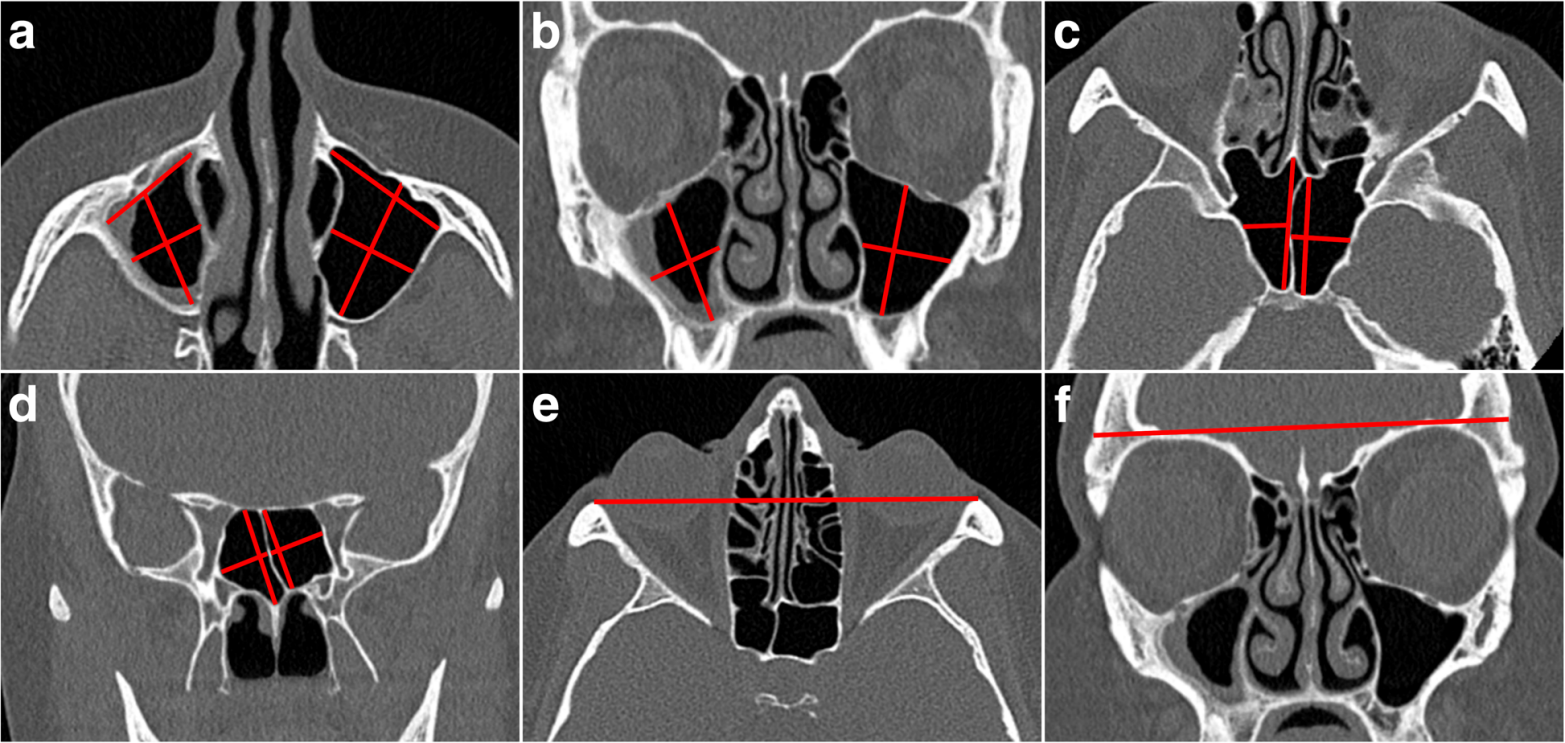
Measurements of the paranasal sinuses and the reference measurements included in calculation of ratio of the mean of maxillary and sphenoid sinus diameters, diameter ratio measurement, (DRM) described in the “Methods” section. The middle widths were measured perpendicular to the depth (the middle longitudinal diameter in the axial plane) or the height (the middle longitudinal diameter in the coronal plane). a Diameter measurements in the suborbital axial plane of the maxillary sinuses, where the sinus area usually is greatest. b Diameter measurements in the coronal plane where the maxillary sinus area is at the greatest usually at the level of the uncinate process. c Diameter measurements in the axial plane of the sphenoid sinuses at the level directly cranial to foramen rotundum. d Diameter measurements in the coronal plane of the sphenoid sinuses at the level of the internal carotic artery crossing the anterior clinoid process. The depth and the height were measured parallel to the septum between the sphenoid sinuses. e Reference diameter measurement in the axial plane: The distance between the lateral orbital walls at the level of the dorsum sellae and the eye lenses. f Reference diameter measurement in the coronal plane: The width of the skull immediately above the orbit in the CT slice at the level of the axial reference measurement
